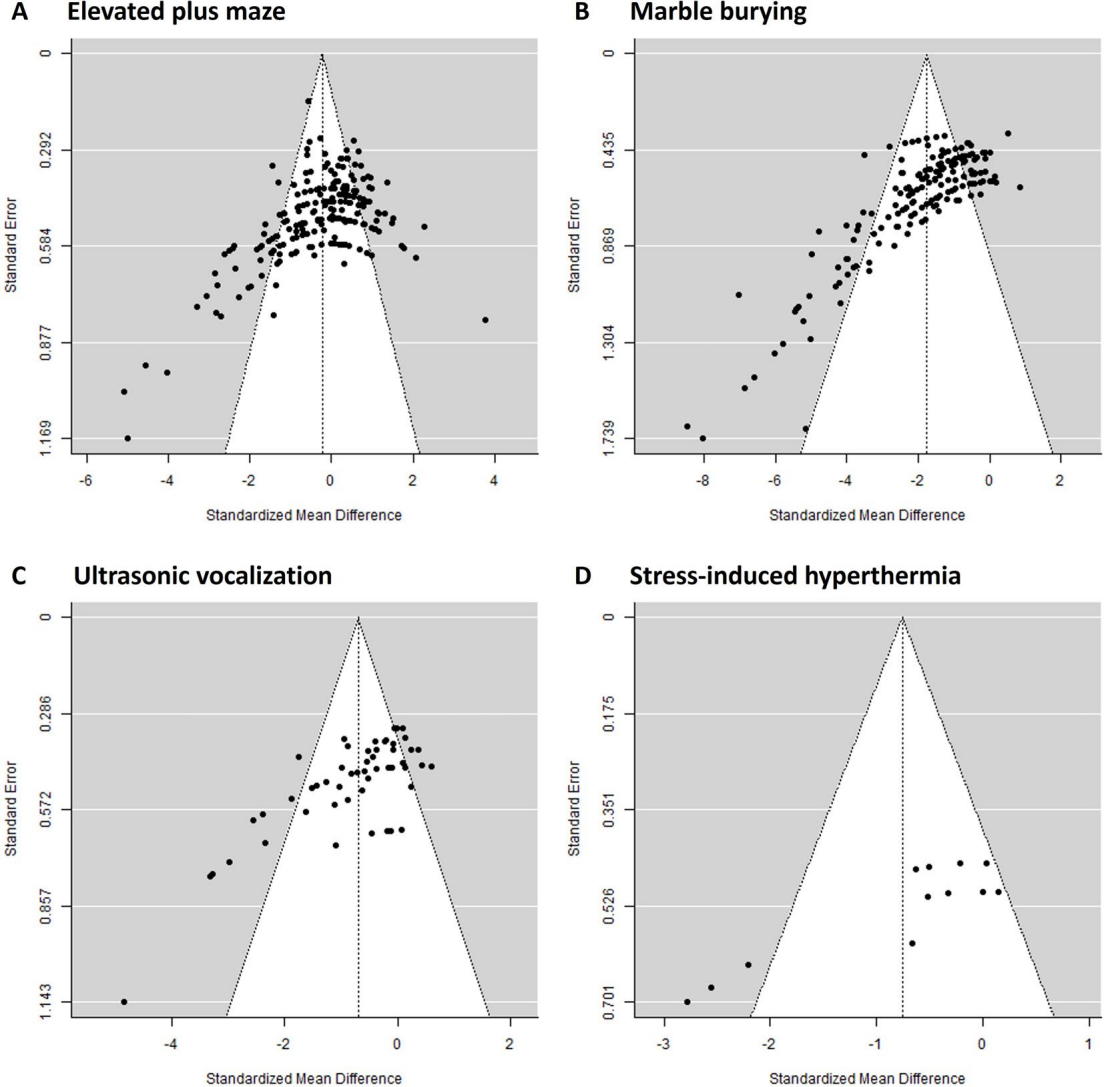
Publication bias visualized by funnel plots for the following unconditioned anxiety tests: (A) elevated plus maze, (B) marble burying, (C) ultrasonic vocalization, and (D) stress-induced hyperthermia. The vertical black dotted line represents the standardized mean difference (SMD) of the overall effect. Negative SMD values favour treatment. The most precise studies are at the top of the plot
